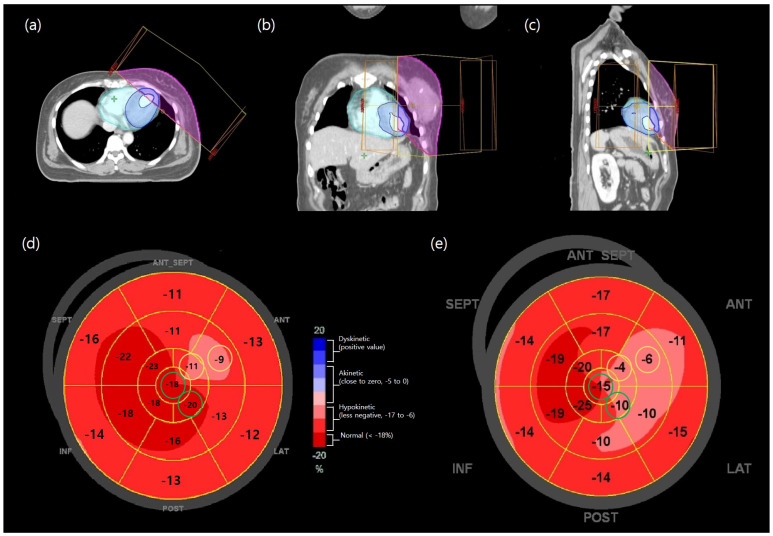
Axial, coronal, and sagittal images of RT planning (a–c) of a 46−year−old woman with left breast cancer and her bull’s eye plot of echocardiography before (d) and 12 weeks after (e) RT completion; the heart is indicated by the cyan translucent area; the blue translucent area indicates the whole left ventricular myocardium; the irradiated area more than 30 Gy is indicated by the pink translucent area; and the irradiated myocardium more than 30 Gy (irradiated myocardium of 30 Gy) is indicated by the blue thick solid line (a–c). The segments representing the normal strain before RT (d, green circles) were changed to hypokinetic (e, green circles) 3 months after RT, and the segments representing the hypokinetic strain (d, yellow circles) were changed to akinetic (e, yellow circles). The changed segments are mainly the apex, apical−anterior, and mid-anterior regions, corresponding to the irradiated myocardium of the 30 Gy.
